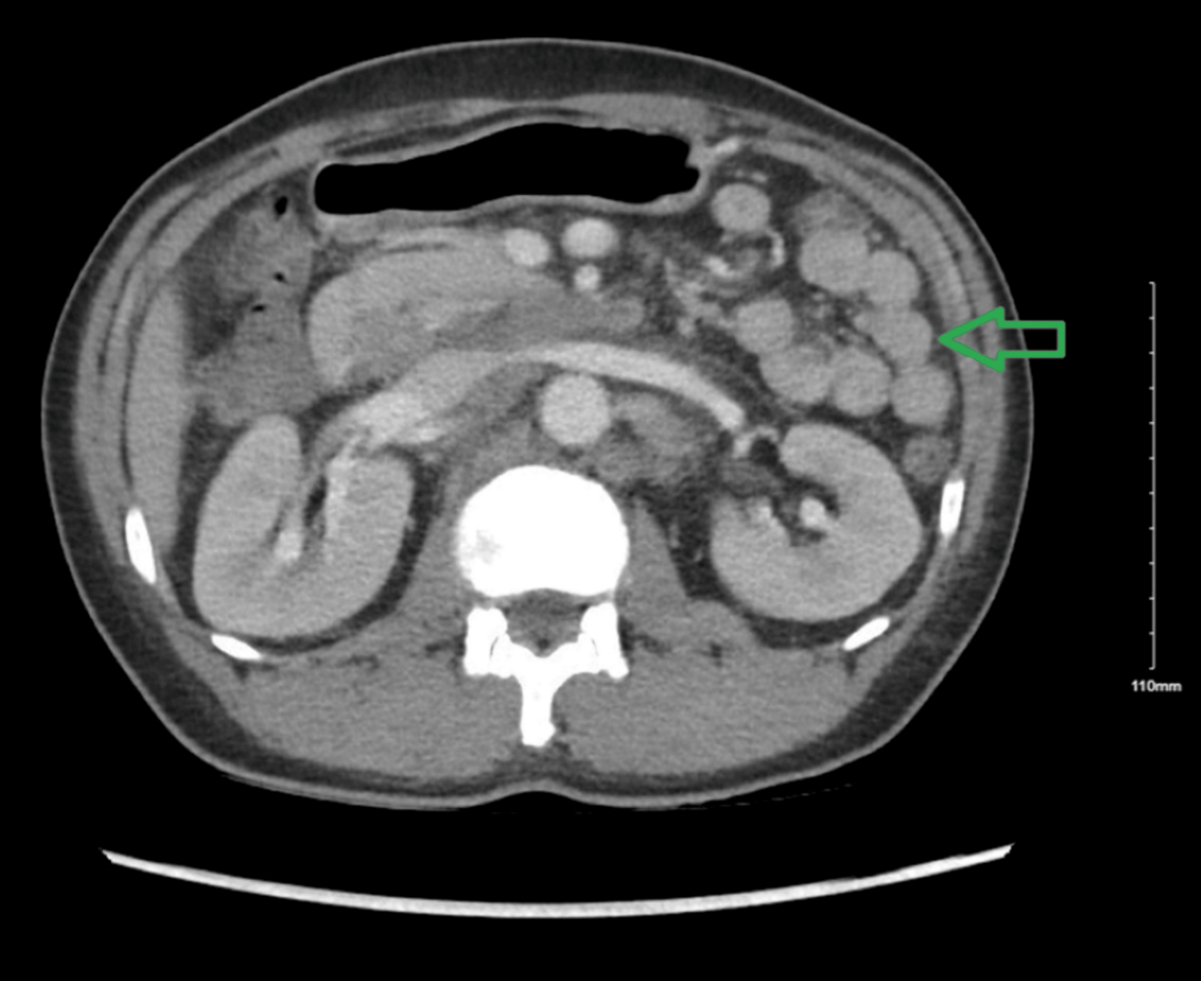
CT abdomen and pelvis without contrast (axial view) Green arrow: lymph nodes CT, Computerized Tomography
